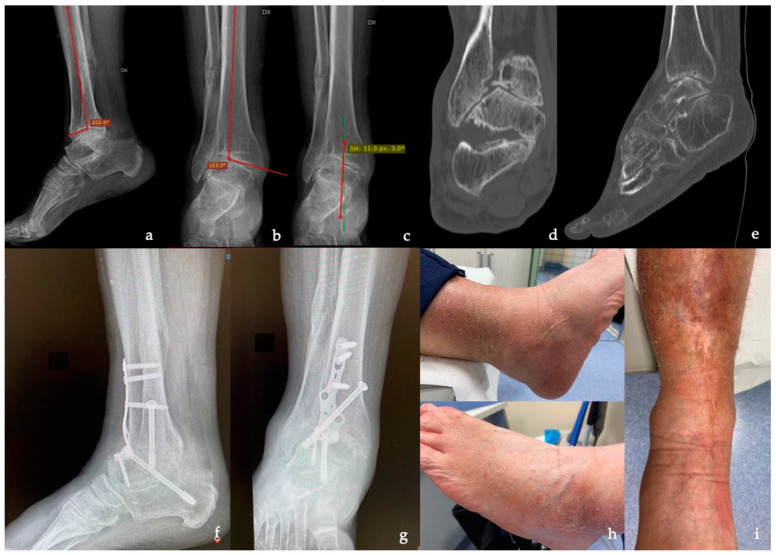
A 55-year-old man with severe osteoarthritis (OA) and deformity of the right ankle and a history of rheumatoid arthritis, diabetes mellitus, and venous insufficiency. (a–c) Pre-operative X-ray in the lateral and anteroposterior view. (d,e) Pre-operative CT showing severe OA of the TT joint. (f,g) Lateral and anteroposterior X-ray at 3 months of follow-up from tibiotalocalcaneal (TTC) arthrodesis with the anterior plate showing complete bone union. (h,i) Clinical image of the soft tissue condition.
